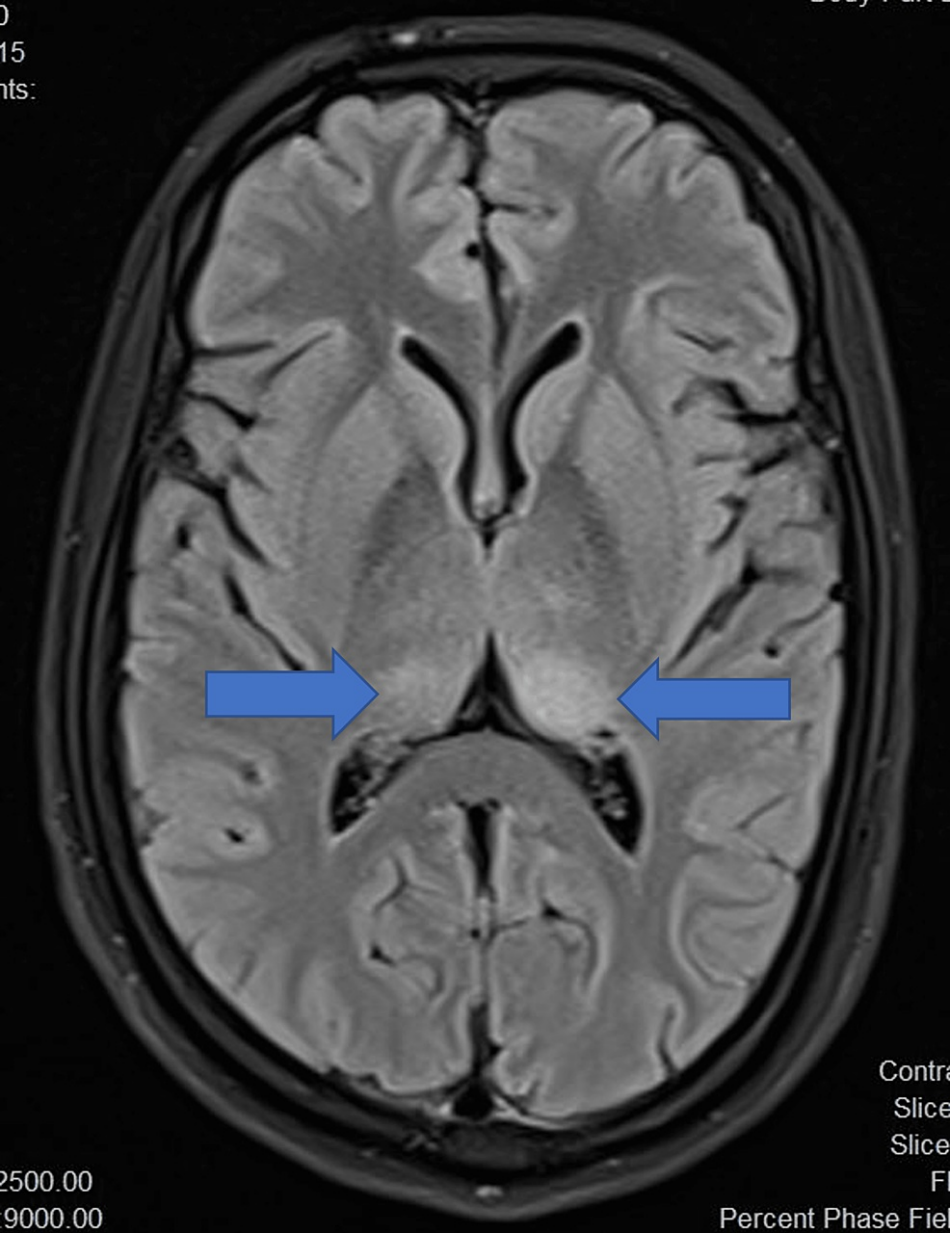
T2 FLAIR axial MRI scan showing bilateral nearly symmetrical hyperintensities in the thalamus FLAIR: fluid-attenuated inversion recovery; MRI: magnetic resonance imaging
